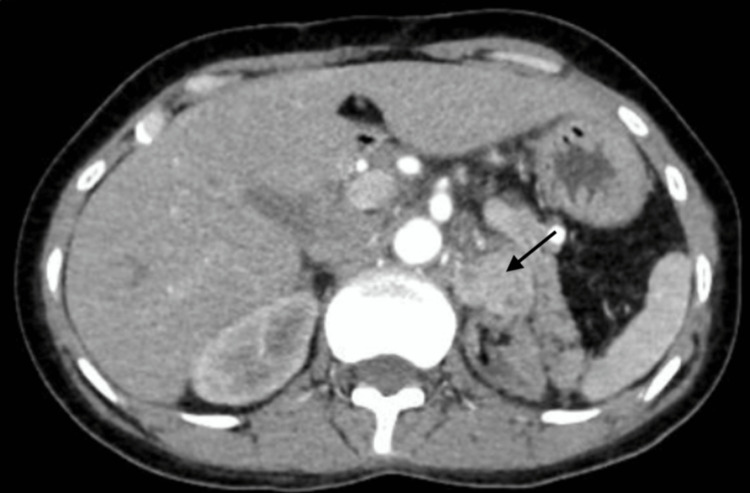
Contrast-enhanced CT scan of the abdomen Showing a left paraaortic mass (arrow) encasing left renal vessels
